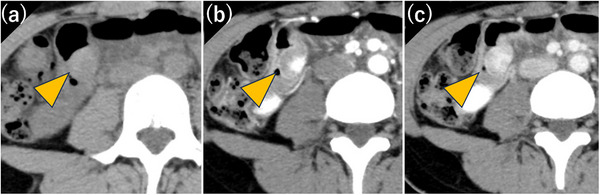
Abdominal computed tomography (CT) findings. (a) Plain CT. (b) Early‐phase contrast‐enhanced CT. (c) Portal venous phase. The tumor (arrowhead) is recognized as a smooth, elevated lesion within the small intestine, showing progressive enhancement.
